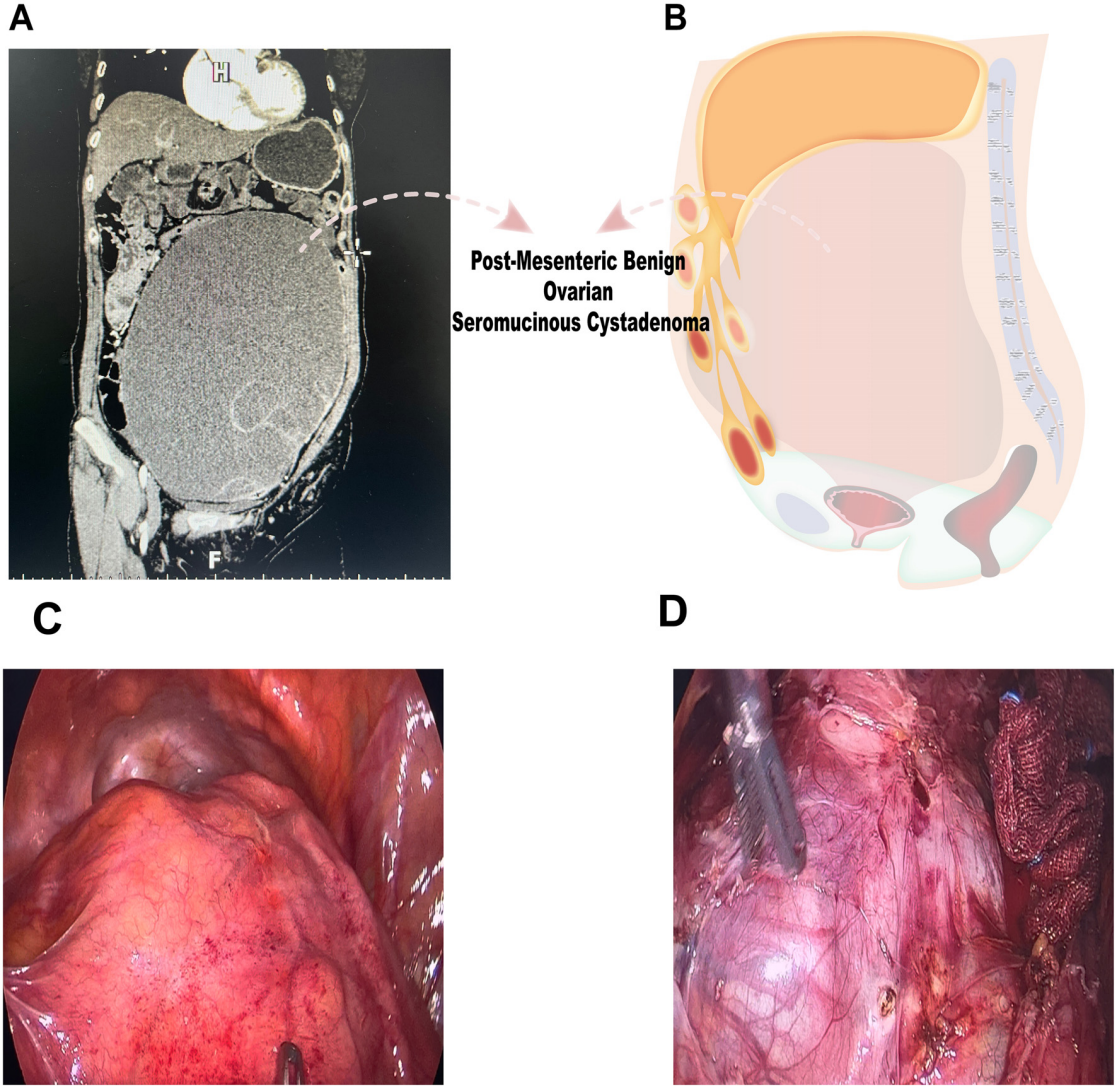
Preoperative imaging examination and intraoperative findings. (A, B) CT imaging demonstrated a substantial lesion within the pelvic cavity, measuring approximately 22.8 cm × 12.4 cm × 30 cm, suggesting a complex internal structure. (C, D) Laparoscopic examination revealed a large cystic mass with high tension, smooth surface, and clear fluid.
